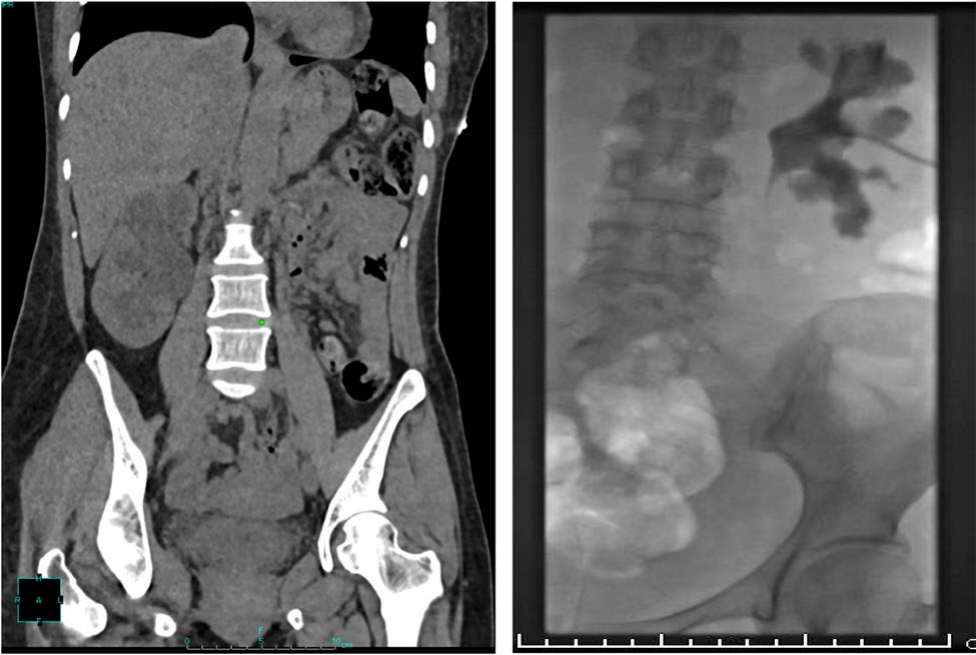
CT scan showing dilated right kidney with hydroureteronephrosis
